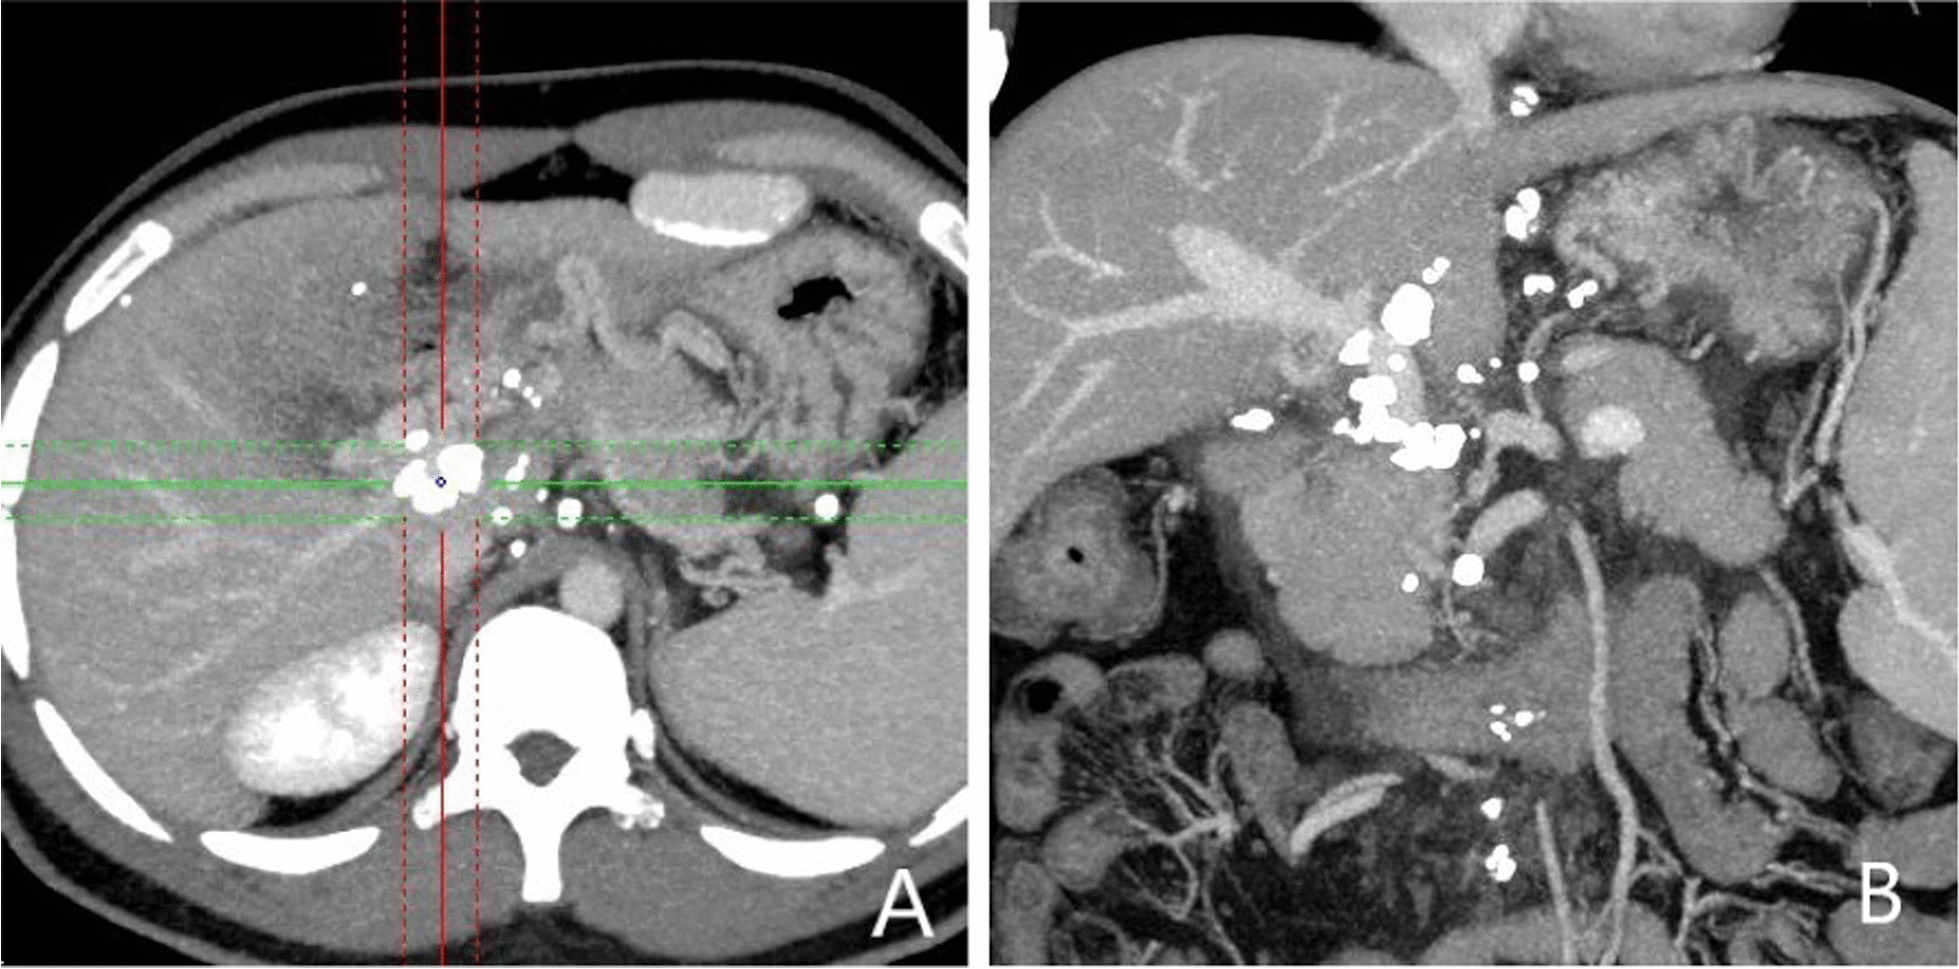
CT axial and coronal views: A and B Esophagogastric fundic varices with multiple lymph node calcifications in the abdominal cavity compressing the portal vein causing portal stenosis
